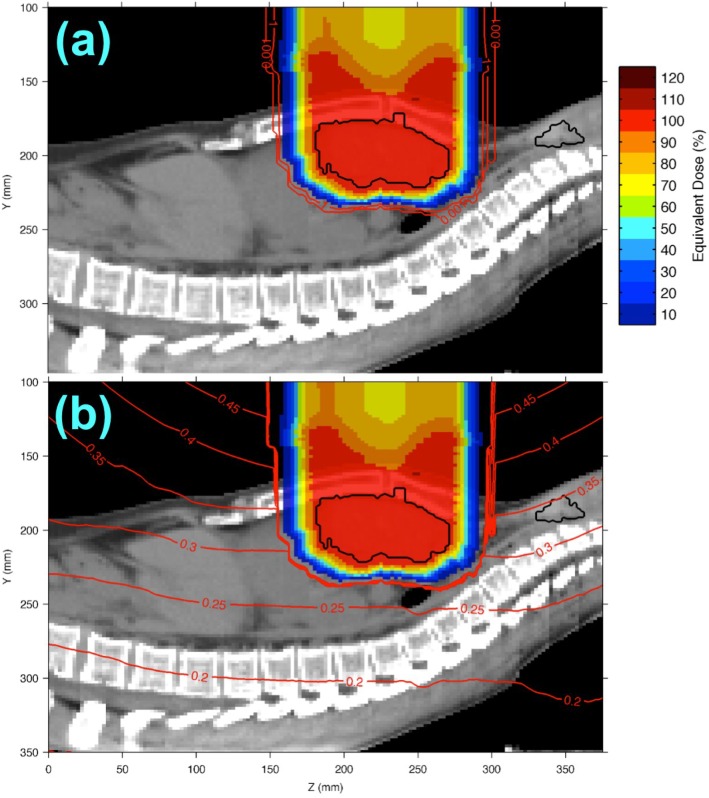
Sagittal equivalent dose planes overlaying a thoracic CT image of the HL patient showing (a) proton equivalent dose and (b) combined proton and neutron equivalent dose. Equivalent dose values are percentages of the prescribed target equivalent dose, *i.e.*, 36 Sv. The mediastinal tumor and healthy thyroid are contoured in black.
